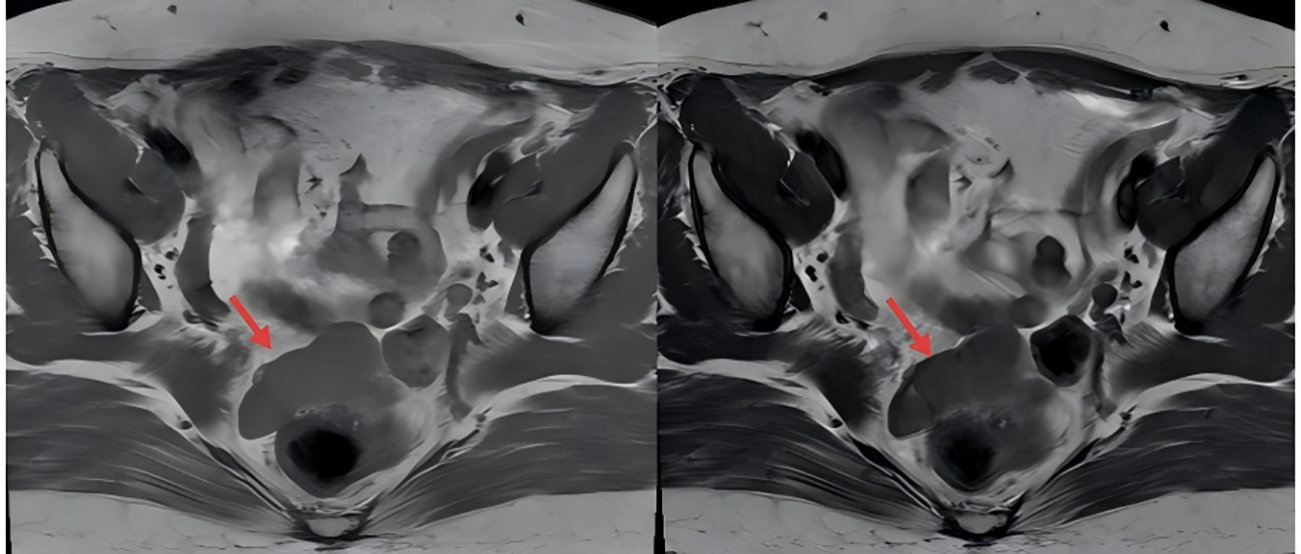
Magnetic Resonance Imaging (MRI) T2-weighted (2024). Red arrows show the same lesions, demonstrating no significant interval change.
